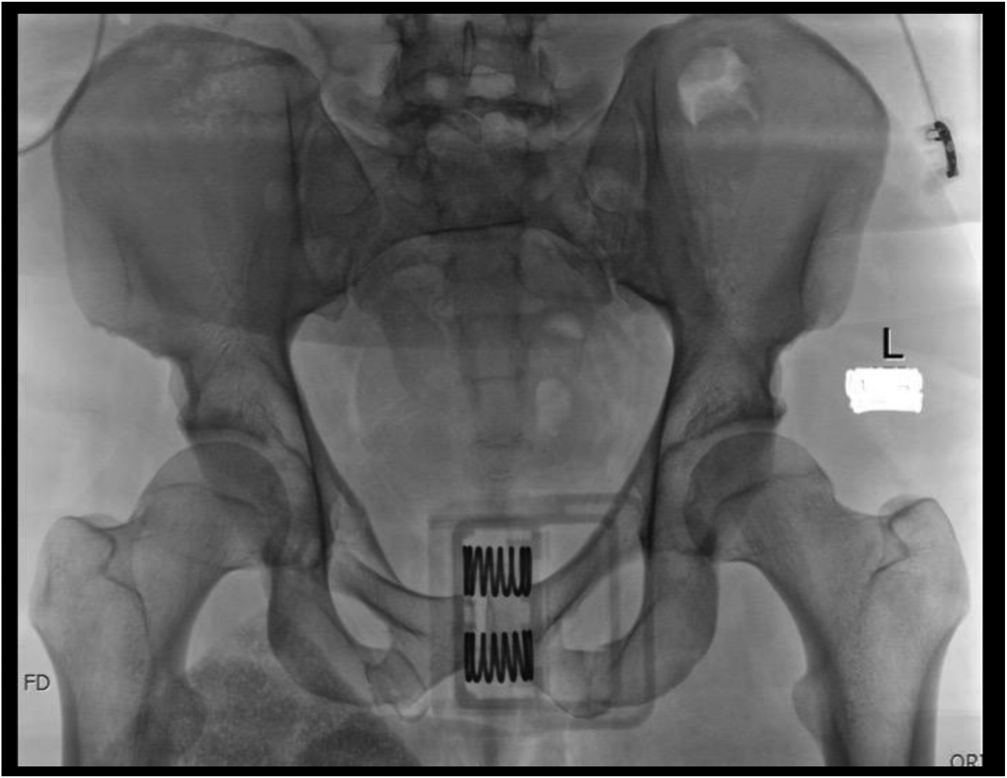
Straddle type fracture-bilateral public rami fractures with bilateral sacral fractures. Initial radiograph on arrival with binder on.
